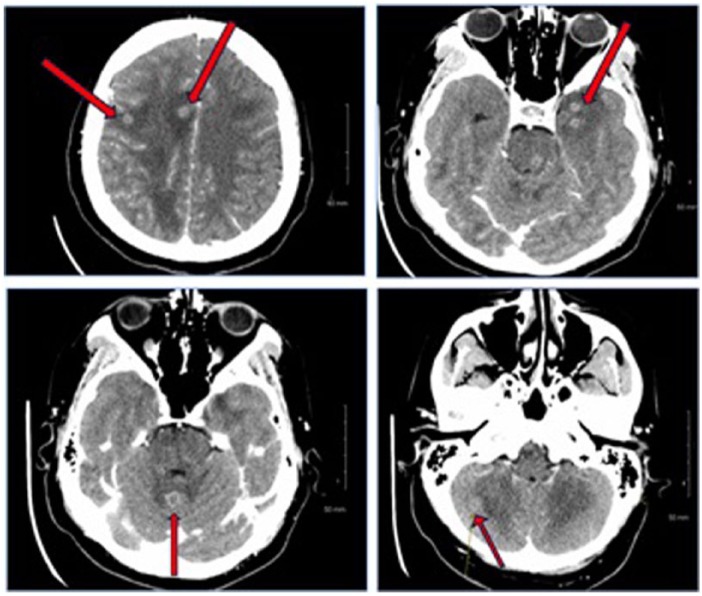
Computed tomography scan with numerous infratentorial and supratentorial tiny
